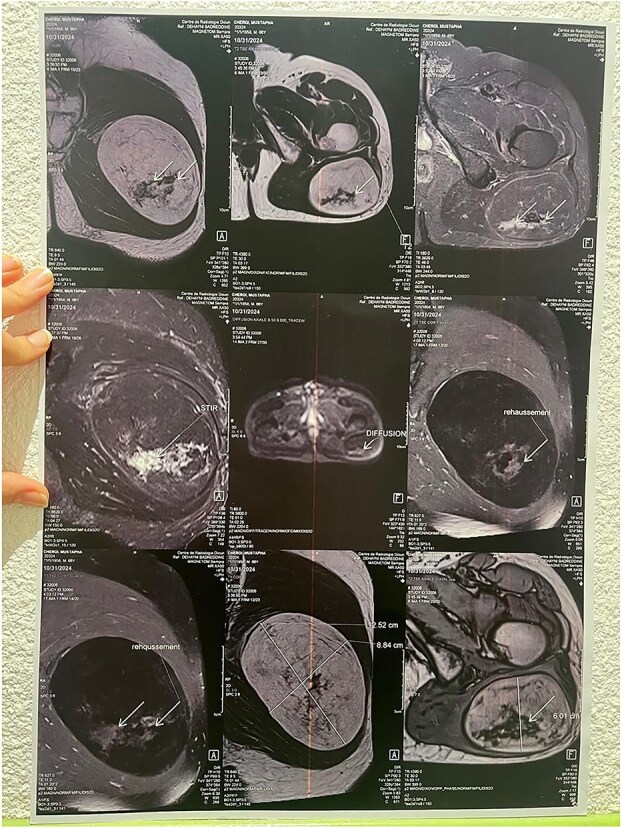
Pelvic MRI (1.5 T) showing the large intramuscular lipomatous mass in the right gluteus maximus. Note thick irregular septations that are STIR-hyperintense, restricted on DWI, and show avid gadolinium enhancement, raising suspicion for WDLPS.
